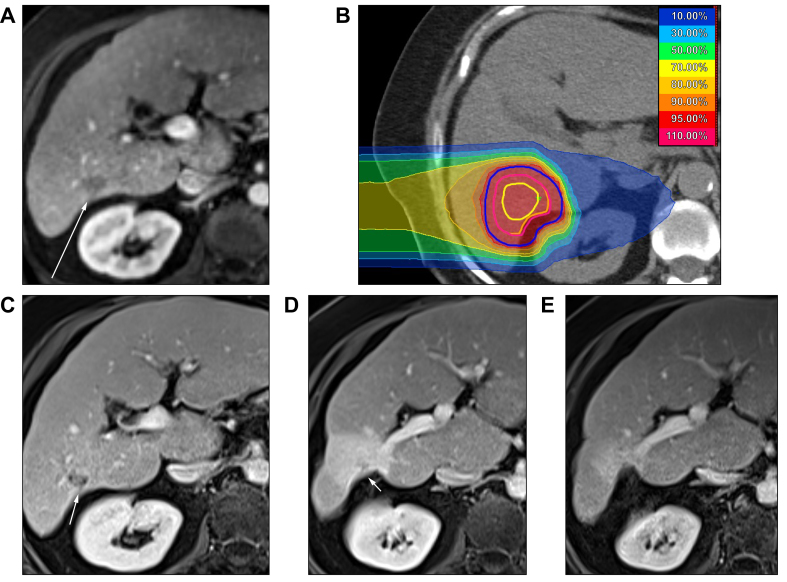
Carbon ion radiotherapy (CIRT) plan and MR images (T1, portal-venous contrast phase) at planning and during follow up. (A) Before CIRT; (B) CIRT plan with isodoses (prescribed dose 42.0 Gy [RBE] = 100.0%). Contours: yellow, GTV; orange, CTV; pink, ITV; blue, PTV. Note the conformal sparing of the adjacent right kidney. (C) One month after CIRT: stable disease. (D) Nine months after CIRT: partial remission and surrounding reaction with focal retraction of the liver capsule. (E) Two years after CIRT: complete remission and continuous retraction of the liver capsule, but decreasing liver tissue reaction. CTV, clinical target volume; GTV, gross tumor volume; ITV, internal target volume; PTV, planning target volume; RBE, relative biological effectiveness.
